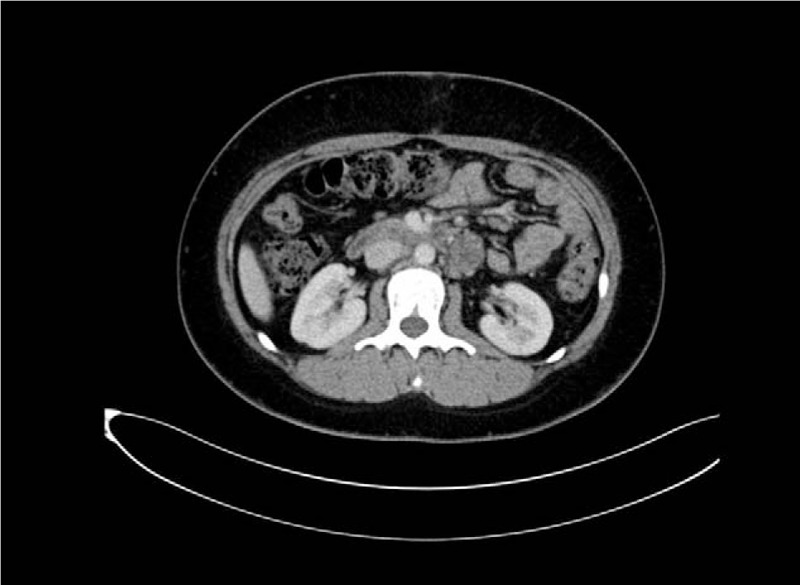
The end-of-treatment follow-up CT scans disclosed no recurrent tumors. CT = computed tomography.
